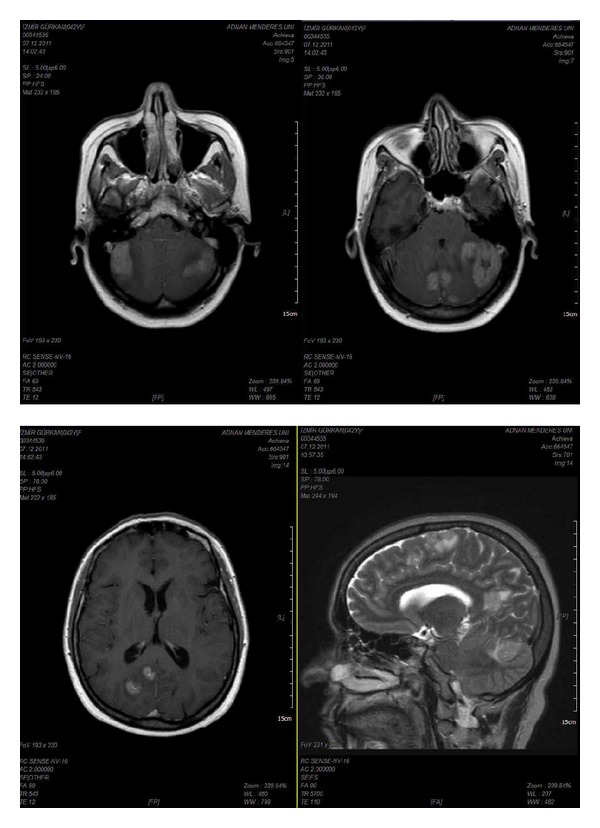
Magnetic resonance imaging scans demonstrating multiple brain lesions.
